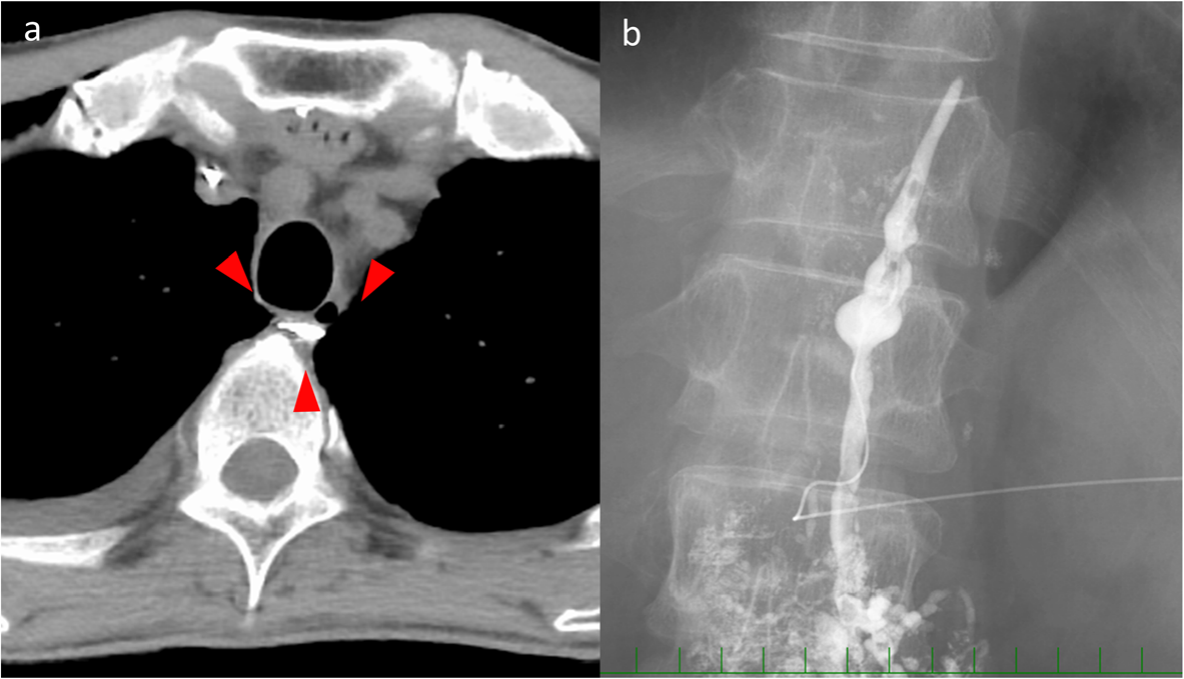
a CT image taken after lymphangiography. Contrast agent leaked from thoracic duct at the level of bifurcation of trachea. b Lymphangiography through the cisterna chyli revealed thoracic duct was dissected in the thoracic cavity
